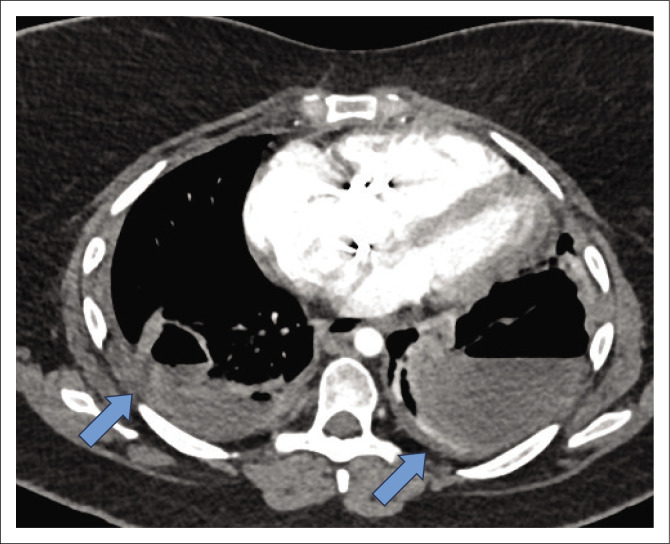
Axial view of computed tomography chest demonstrating bilateral lung abscesses, most notably on the left.
